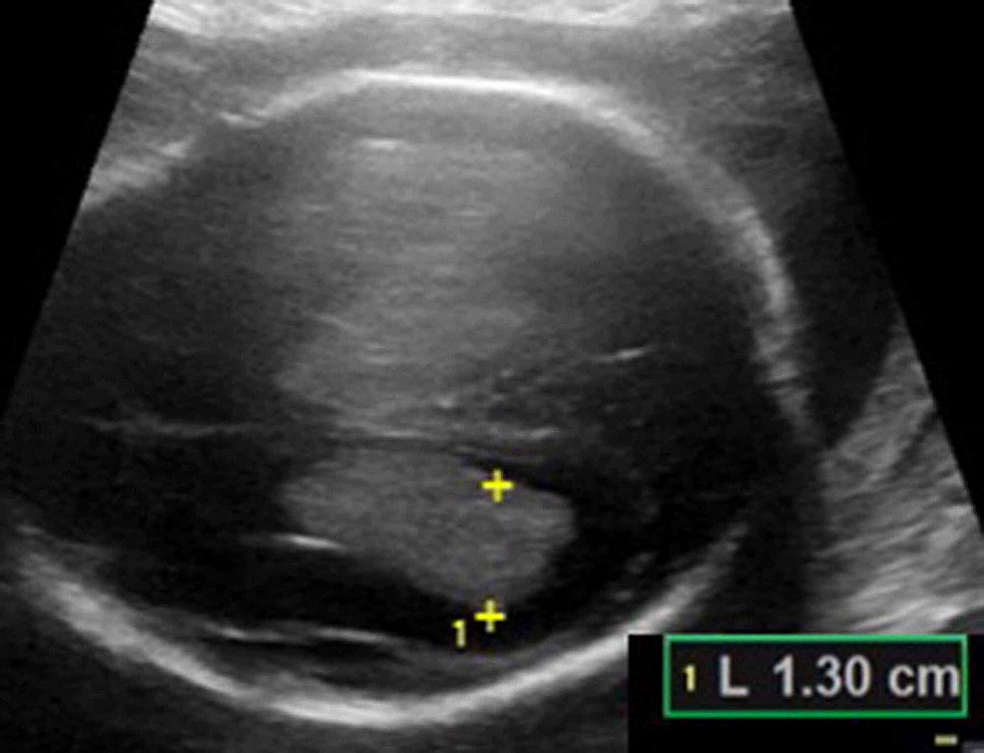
Transverse sonographic image of the fetal brain at 20 weeks 6 days, obtained by transabdominal ultrasound Enlarged bilateral choroid plexus, with associated ventriculomegaly is seen; left lateral cerebral ventricle measures 13 mm in atrial diameter.
